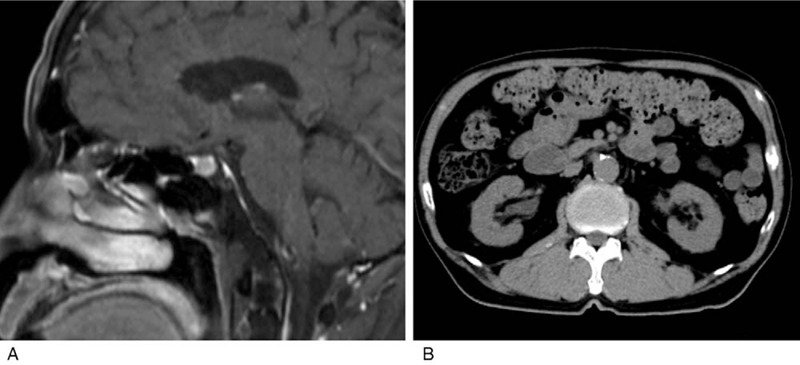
Follow-up imaging 2 months after the diagnosis of IgG4-related disease. A: Magnetic resonance imaging showed improvement of the pituitary lesion. B: Computed tomography showed improvement of the retroperitoneal lesion.
